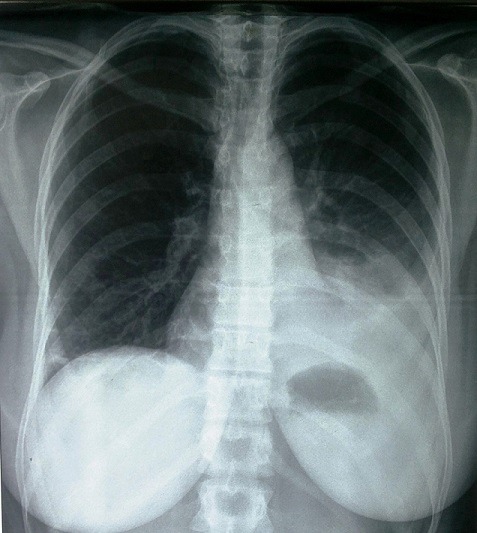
Radiographie thoracique de face montrant une pleurésie gauche de moyenne abondance
